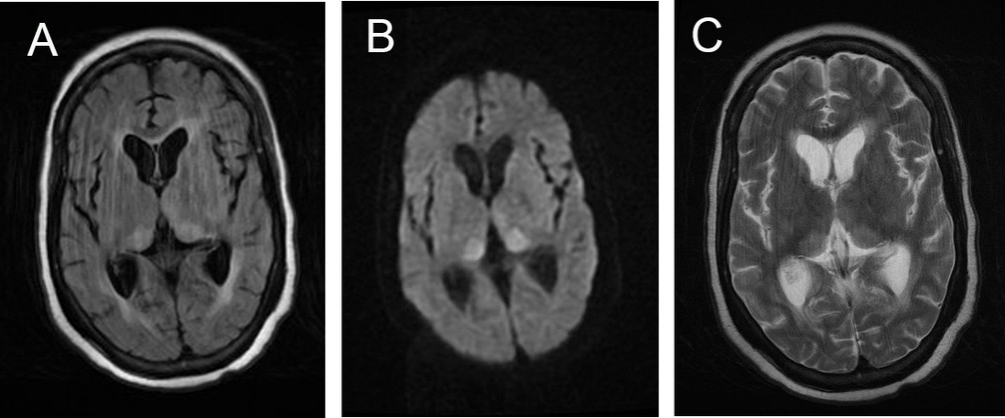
MRI in a patient with autoimmune encephalopathy due to NMDA antibodies is similar to the scans seen in vCJD. Note pulvinar sign on FLAIR (A) and DWI (B). The ADC scan did not confirm restriction (C). There is minor periventricular high signal in the FLAIR sequence (A). ADC, apparent diffusion coefficient; DWI, diffusion-weighted images; NMDA, N-methyl-D-aspartate antibodies.
